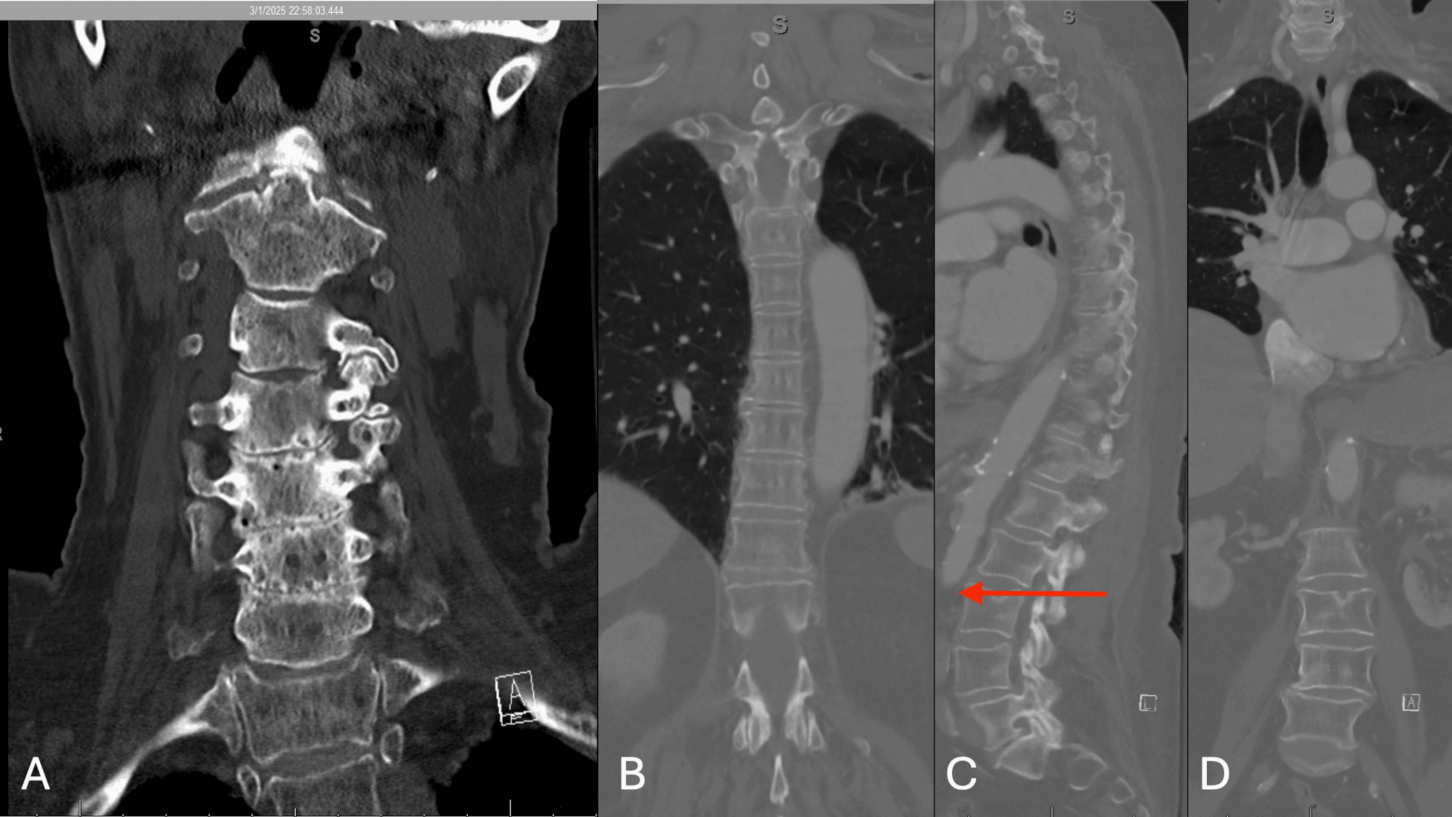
Computed tomography of the cervical, thoracic, and lumbar spine, without evidence of acute spinal fracture, dislocation, or injury. A: Coronal section of cervical spine computed tomography without acute spinal abnormality; B: Coronal section of thoracic spine computed tomography without acute spinal abnormality; C: Sagittal section of lumbar spine on computed tomography without acute spinal abnormality, though evidence of aortic occlusion (red arrow) better demonstrated on angiography in Figure 3; D: Coronal section of the lumbar spine on computed tomography without acute spinal abnormality.
